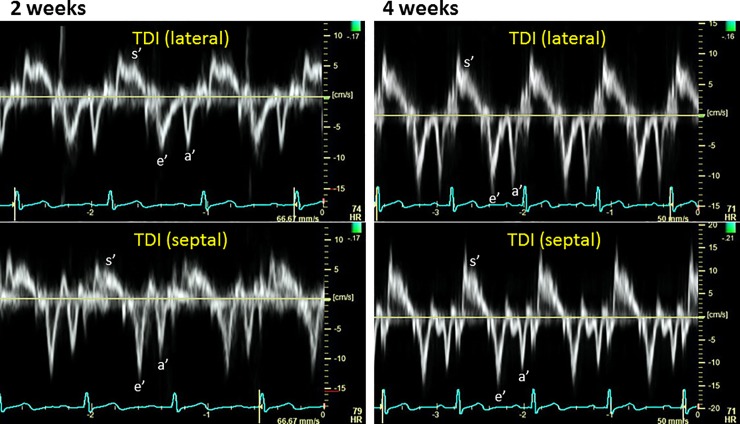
Tissue Doppler profiles for the lateral (top) and septal (bottom) corners of the mitral annulus, for the patient as in Fig 3.
